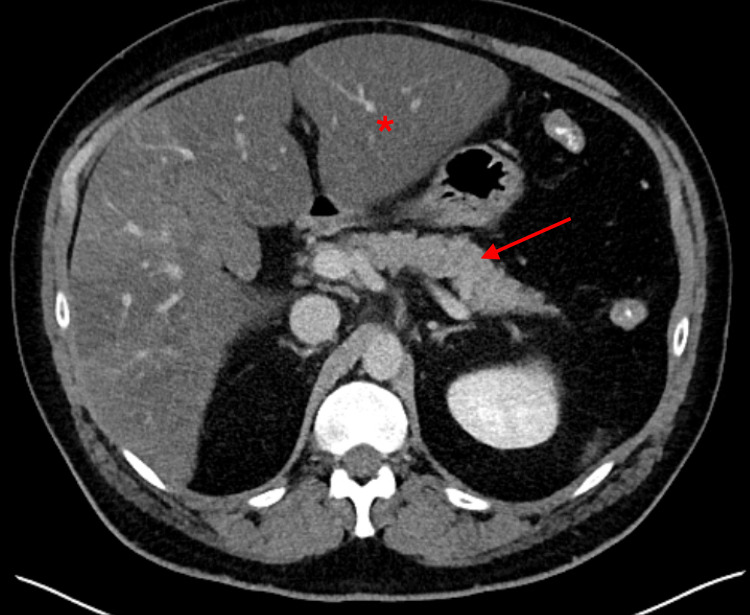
Initial CT revealing liver hypoattenuation consistent with hepatic steatosis (asterisk) and normal appearance of pancreas (arrow)
